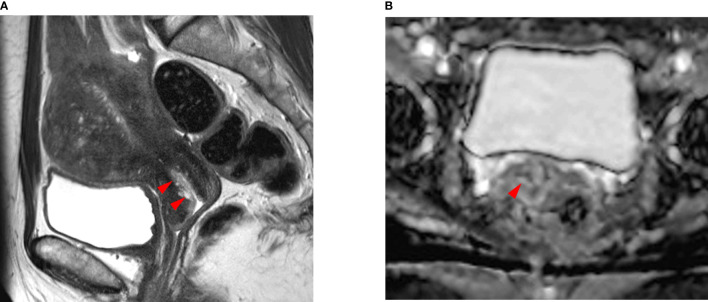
A 48-year-old woman with endocervical adenocarcinoma. (A) The T2-weighted sagittal MR image shows no tumor in the uterine cervix. The red arrows indicate a poorly demarcated cystic mass, which was preoperatively interpreted as normal endocervical glands. (B) The apparent diffusion coefficient (ADC) axial image shows no focal lesion with low ADC values in the cervical canal (red arrowhead). However, the pathologic report confirmed that there was a residual tumor in the endocervical canal. The tumor size was measured as 2.0 × 1.5 cm and the depth of stromal invasion was 0.4 cm in a 1.3-cm cervical wall. It was well-correlated with the endocervical lesion in (A). Tumor invasion to the lymphovascular space, vagina, and parametrium and lymph node metastasis were all negative.
